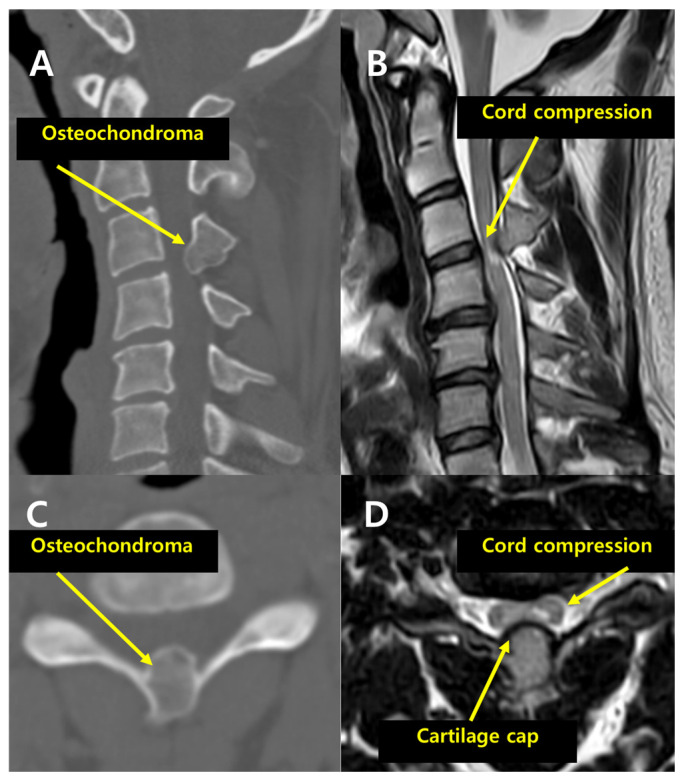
Preoperative imaging of the cervical osteochondroma. (A) Sagittal computed tomography showing a bony lesion (arrow) arising from the base of the C3 spinous process. (B) Sagittal T2-weighted magnetic resonance imaging demonstrating compression of the cervical spinal cord (arrow) by the lesion, with associated cord signal change. (C) Axial computed tomography at the C3 level showing the osteochondroma (arrow) arising from the base of the spinous process and extending into the vertebral canal. (D) Axial T2-weighted magnetic resonance imaging at the C3 level confirming compression of the spinal cord (arrow) by the lesion and demonstrating a thin cartilage cap (arrow).
